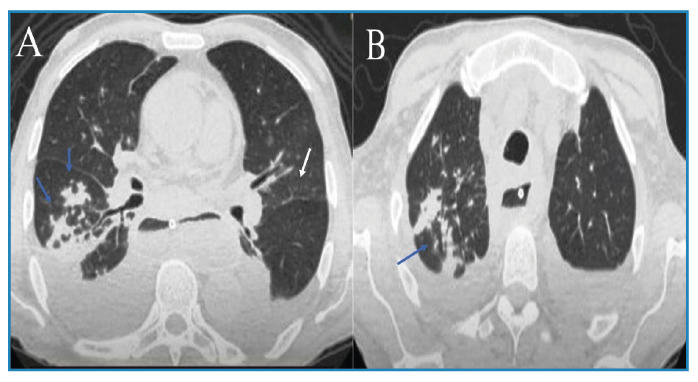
Axial plane thoracic computed tomography images show bilateral pleural effusion, ground-glass opacity in the left lung (white arrow on image A), and a tree-in-bud pattern in the right lung (blue arrows on images A and B).
